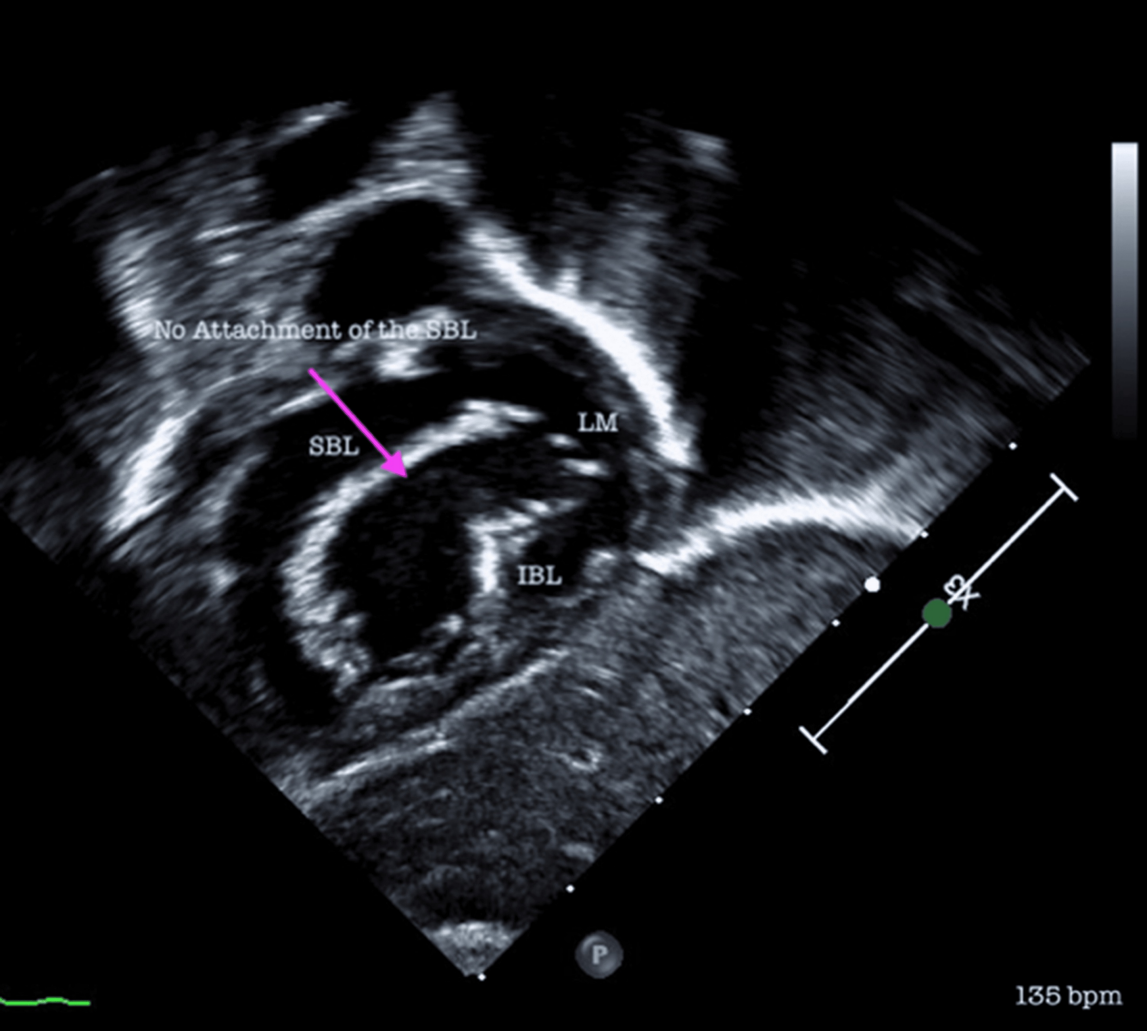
Ventricular septal defect Rastelli TYPE C – no superior leaflet bridging attachment. A subcostal view in transthoracic echocardiography shows a common atrio-ventricular valve with five leaflets and no attachment of the superior bridging leaflet (Atrioventricular septal defect Rastelli type C). SBL: Superior bridging leaflet; LM: Left mural leaflet; IBL: Inferior bridging leaflet
